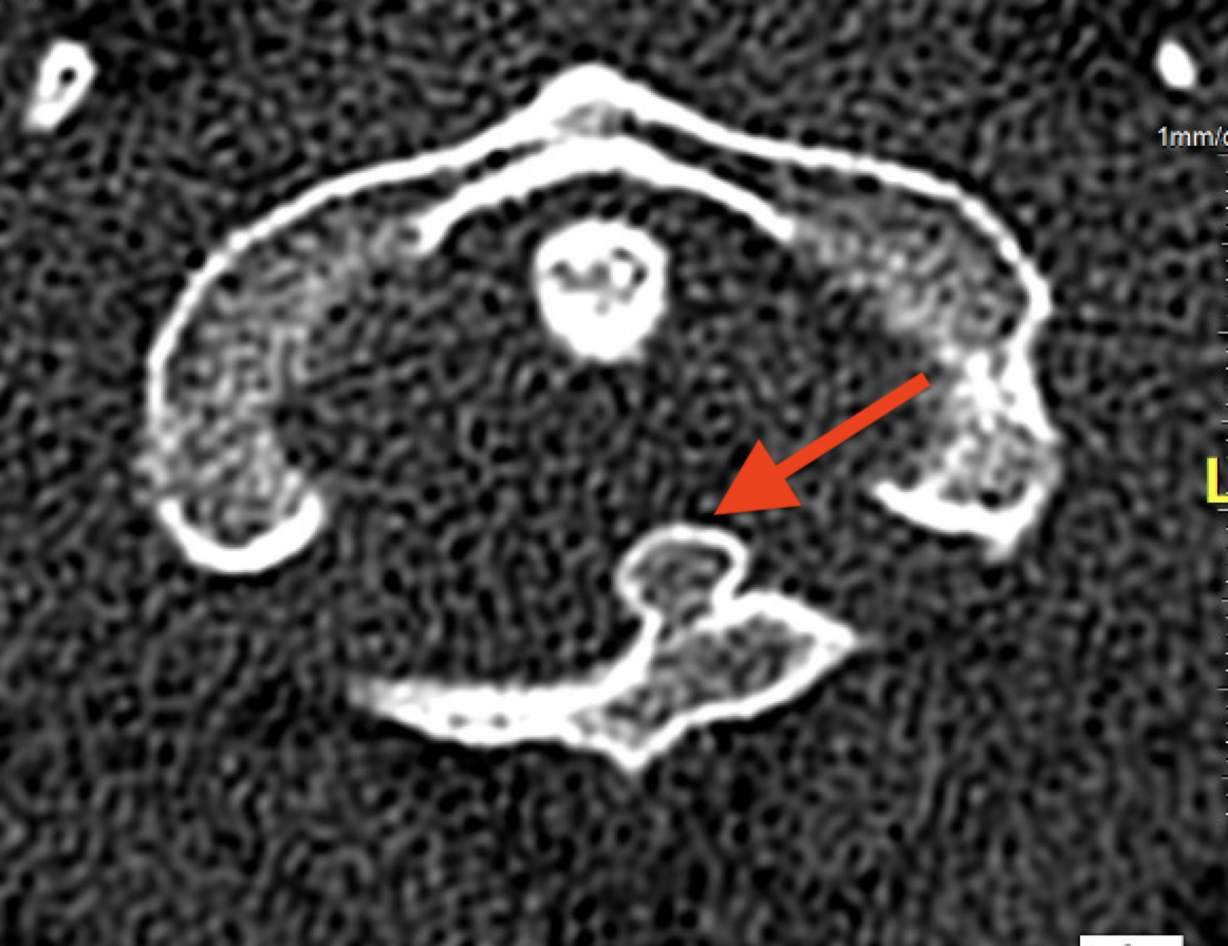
Cervical CT axial view demonstrating a pedunculated osteochondroma on the posterior arch of C1
